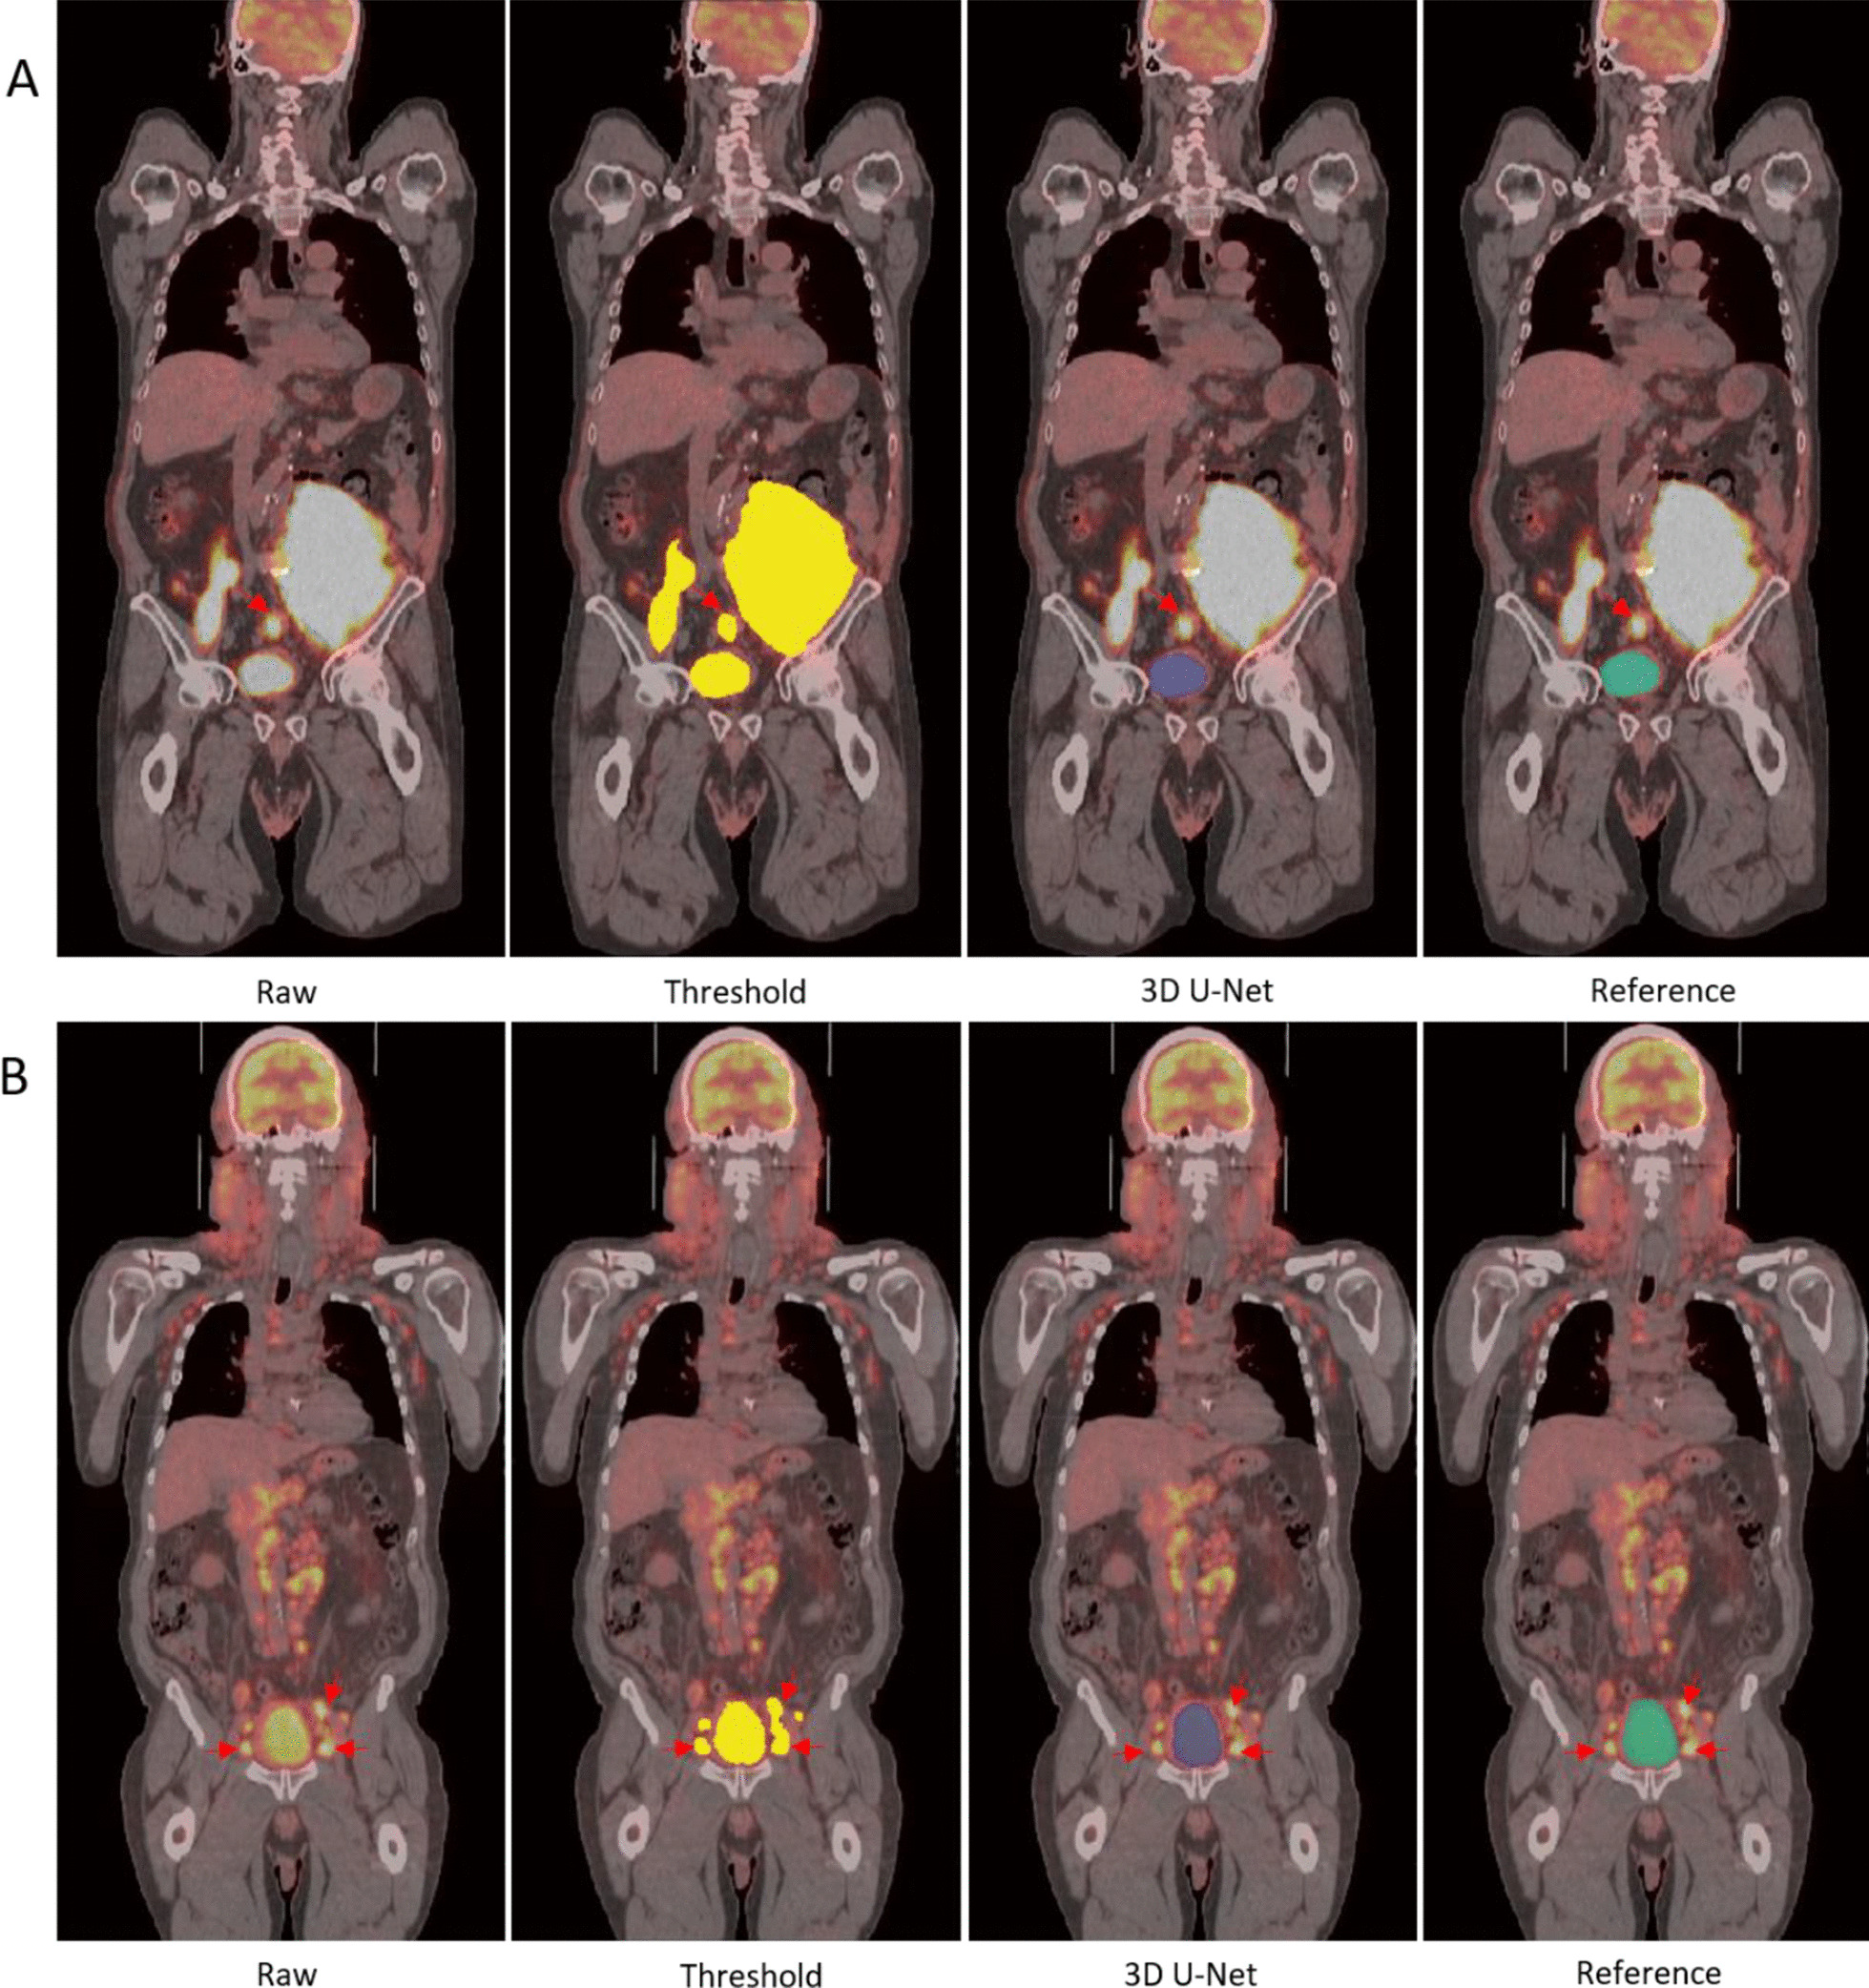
Example of bladder segmentation with tumor nearby indicated by red arrows on PET/CT image. Threshold based segmentation (yellow), automated segmentation (blue) and reference segmentation (green) are present
